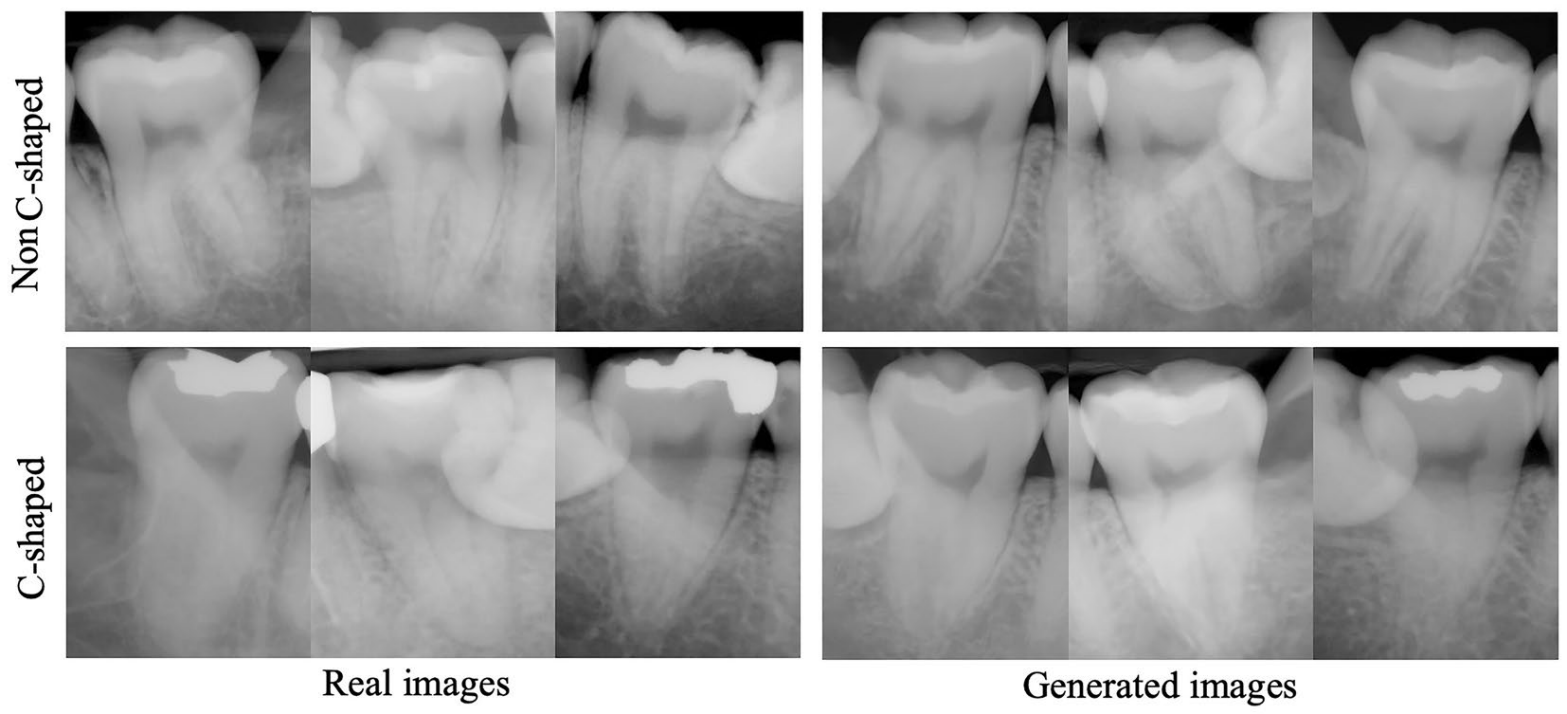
Examples of qualitative comparison between the original periapical images and GAN synthesized images having C-shaped or non C-shaped canal configuration. Some generated images show lower precision in the apical area with features such as fuzzy appearing apexes or periodontal ligaments, and less prominent root canals.
